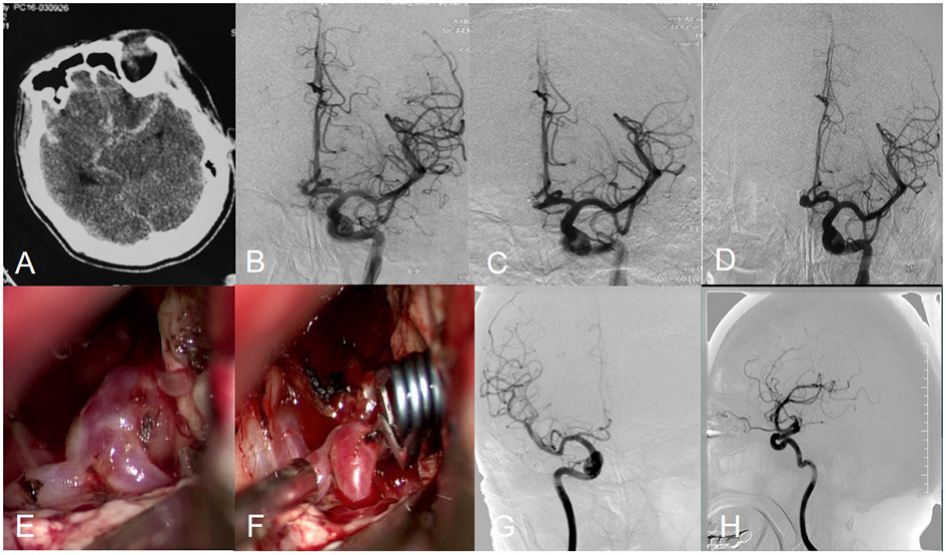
Angiograms obtained in Patient 12, a 53-year-old female with an anterior communicating artery aneurysm. CT scan showed SAH (A). Preembolization angiogram (B). Postoperative arteriogram demonstrating complete obliteration of aneurysm (C). Follow-up angiogram showed aneurysm recurrence (D). Intraoperative photograph showed aneurysm clipping (E,F). Postoperation angiogram showed aneurysm was clipped completely (G,H).
